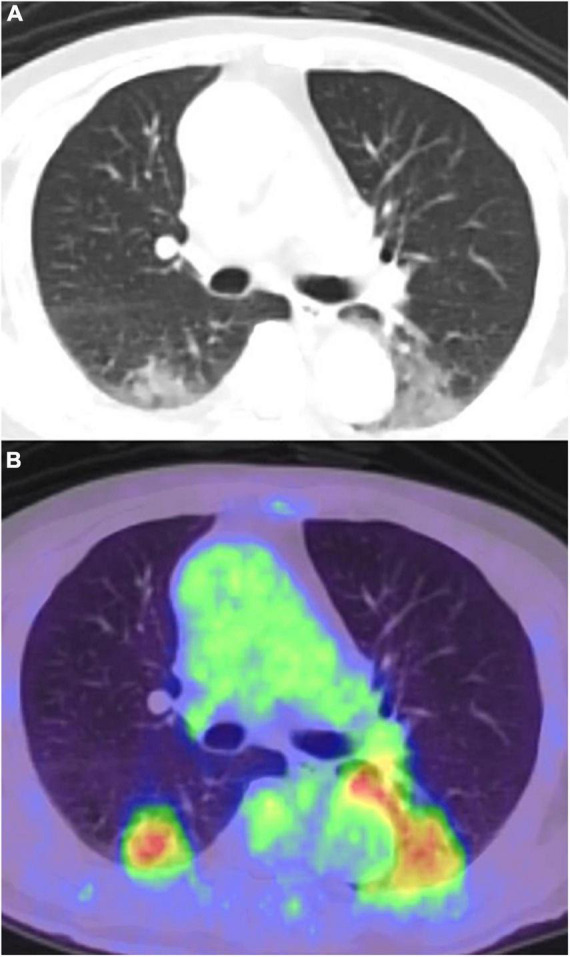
Pneumonia caused by bronchoscopy performed a day before the FDG-PET/CT, misidentified as COVID-19 pneumonia. (A) CT image, (B) PET/CT image.
